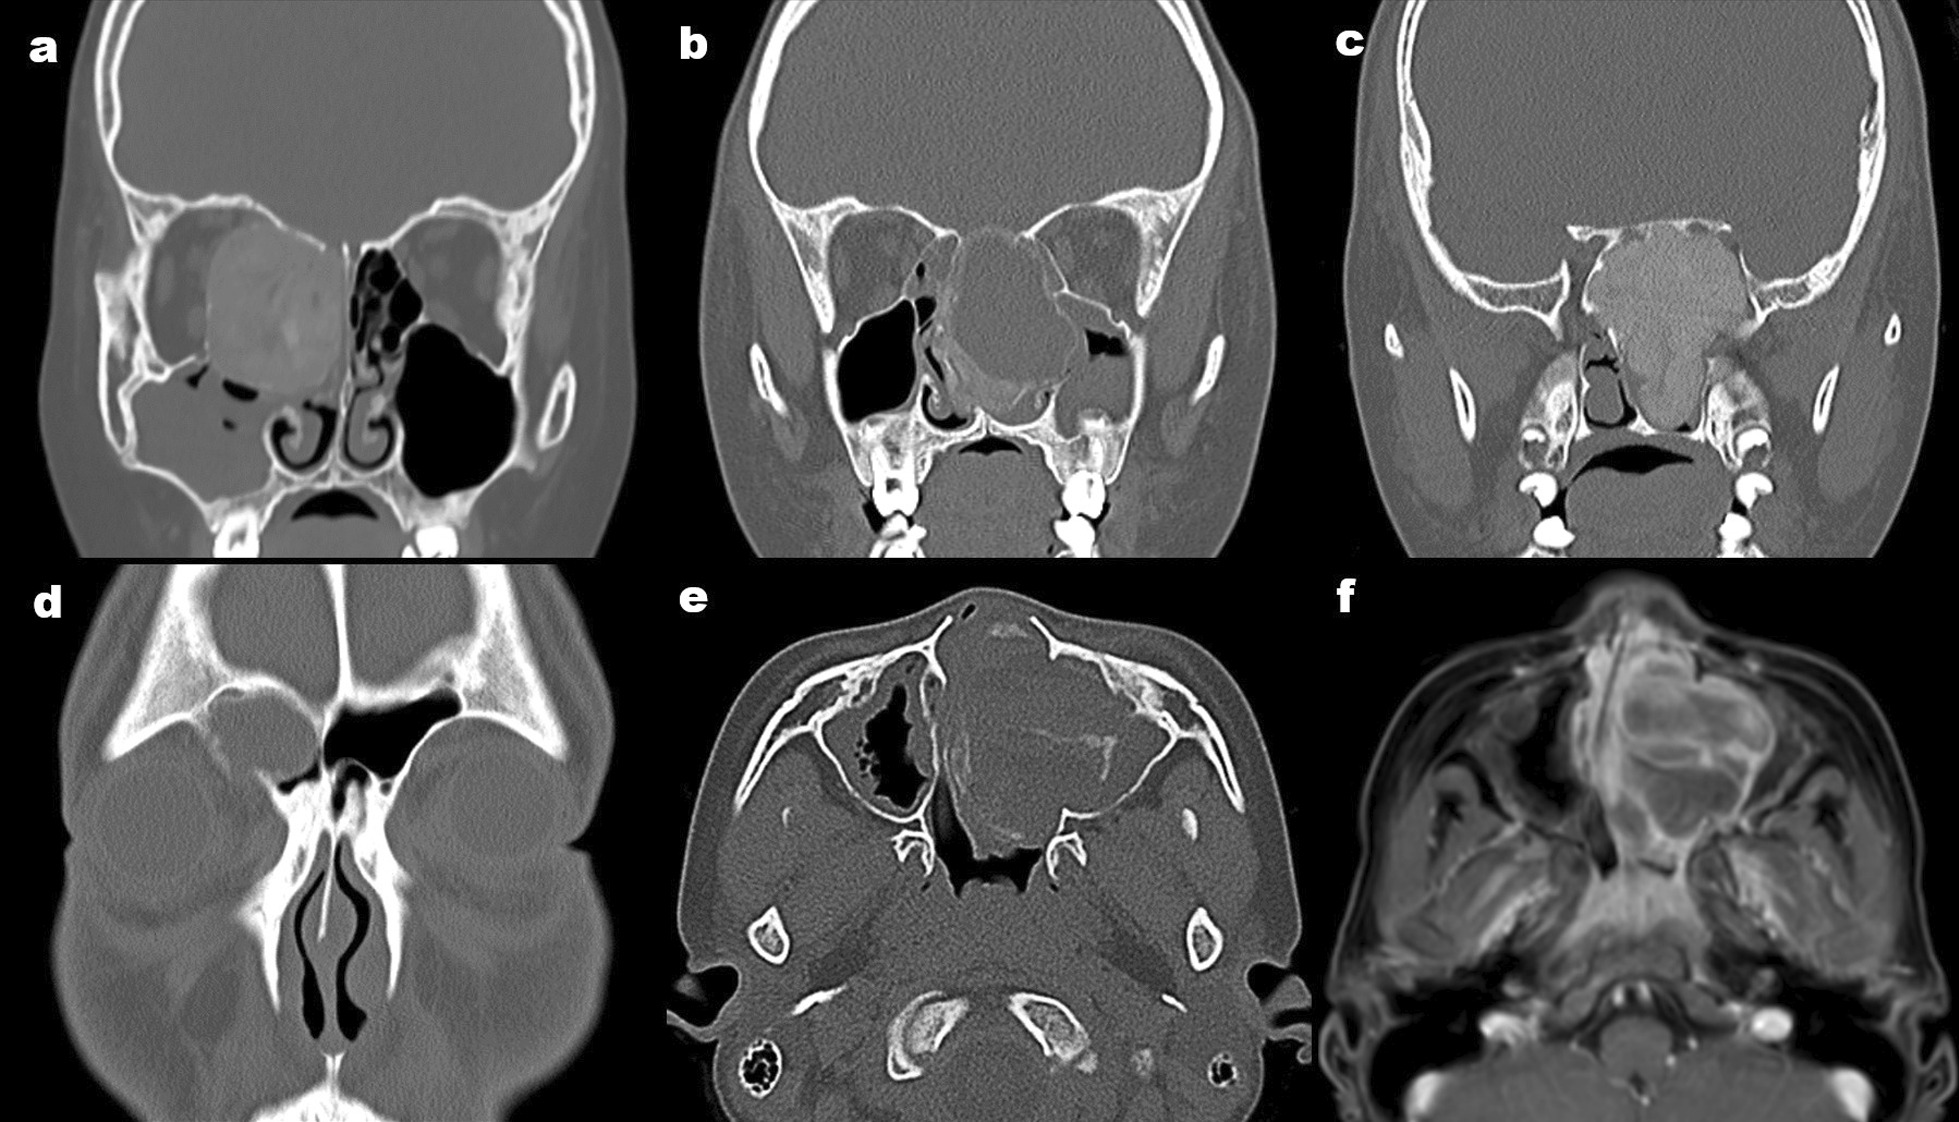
Preoperative coronal computed tomography of the nasal sinus (a–d), preoperative image of juvenile ossifying fibroma (OF) with an aneurysmal bone cyst (e), and enhanced magnetic resonance imaging of an aneurysmal bone cyst (f) in children with OF. a Tumour with orbit involvement, b anterior skull base involvement, c tumour with sphenoid and sella turcica involvement, d tumour with right frontal sinus involvement, e multifocal cystic lesion with peripheral bone shell formation, f more obvious enhancement of the cyst wall and intracapsular septum of the lesion
